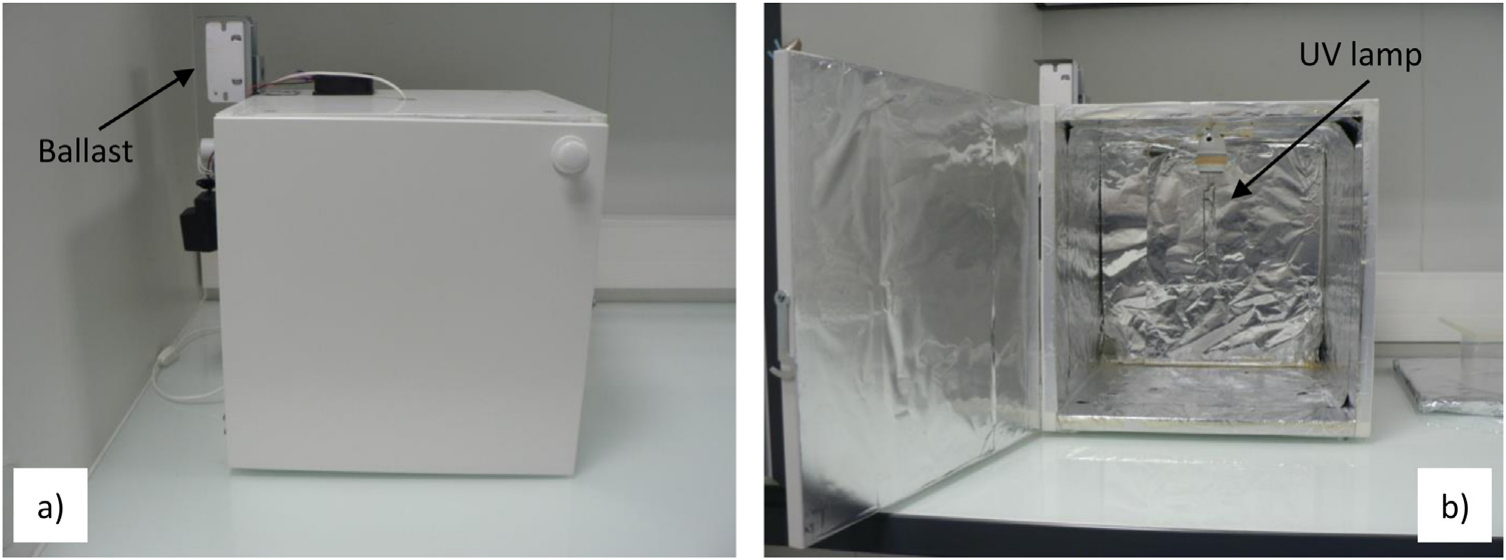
Home-made UV irradiation chamber, lined inside of aluminum foil, equipped with UV lamp (after light bulb was removed) and a ballast for lamp operating. (a) Outside view, (b) Inside view.•Ultrasonic bath (Branson®, MT 8800-E)•Chemical reagents-NaN3 (Sigma-Aldrich®, ReagentPlus)-HCl (VWR®, analytical grade)-HCl (Laboratory distillation, ultrapure grade)-Decon90 (Decon®, Alkaline detergent)•Recipients (Fig. 2)-For field sampling: 20 L High Density PolyEthylene (Nalgene®, HDPE) jerrycans and 500 mL PolyCarbonate (Nalgene®, PC) bottles-For sterilization experiments: 120 mL Borosilicate Glass (Fisherbrand®, BG), PolyEthylene Terephthalate (Thermo Scientific®, PET) or Fluorinated Ethylene-Propylene (Thermo Scientific®, FEP) bottles
